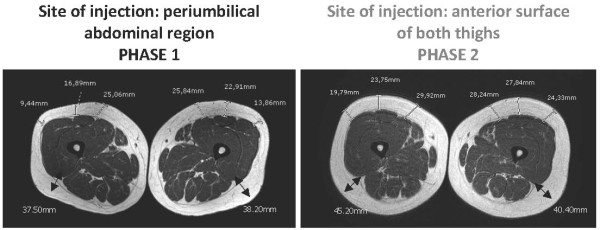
MRI scans of the thighs of patient 1 when injecting pegvisomant (10 mg/day) subcutaneously in her abdomen (Phase 1) and thighs (Phase 2).
